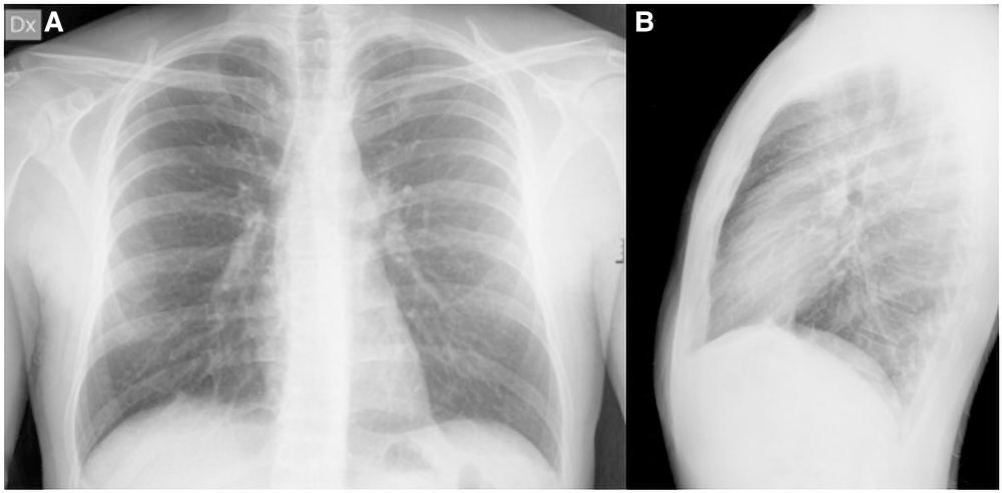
Patient’s ER chest X-ray in antero-posterior (*A*) and latero-lateral view (*B*).
